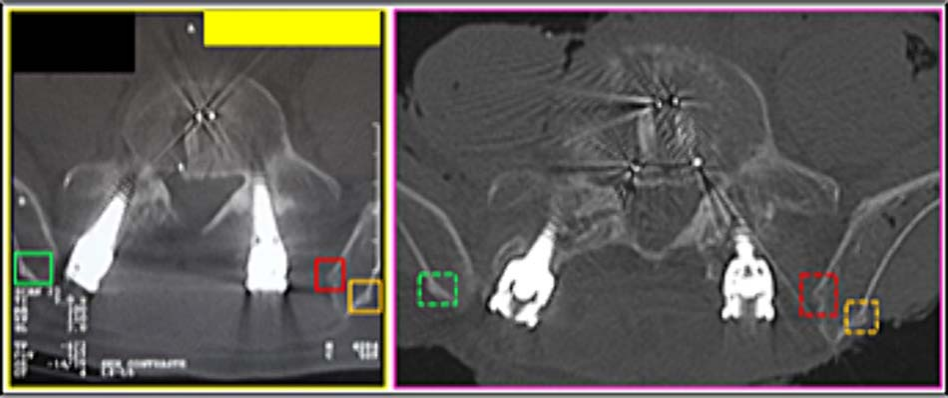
L5 vertebral body. Comparison between the axial slices of the antemortem (left) and postmortem (right) CT scans. Consistent elements.
